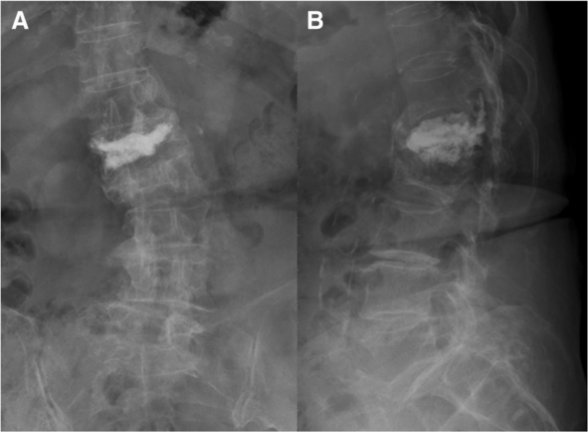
A 76-year-old woman who underwent L1 PV sustained progressive intractable back pain with bilateral lower limb numbness and weakness. The anteroposterior (A) and lateral (B) radiographs revealed cement leakage into the spinal canal and L2 adjacent fracture.
